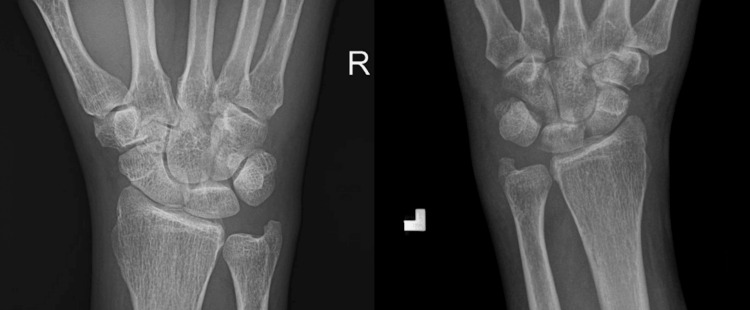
X-rays of left wrist (left) and right wrist (right) on day 0 Bilateral wrists demonstrated diffuse soft tissue swelling around the wrist and dorsal hand. A definite fracture was not identified
